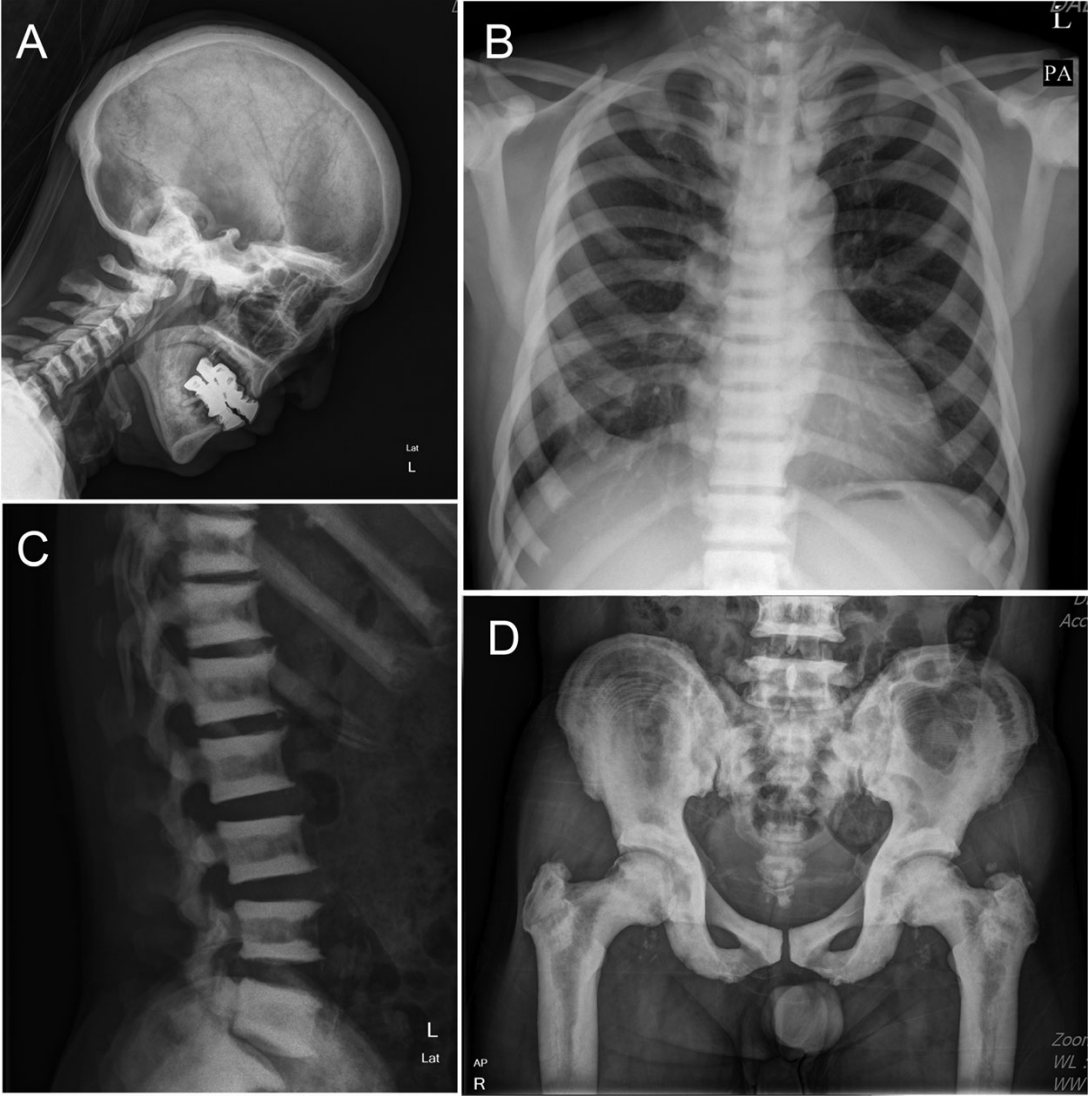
Skeletal radiographs reveal generalized symmetric increases in bone mass. (*A*) The cranium shows a thickening of the skull base. (*B*) Chest postero‐anterior radiograph shows the stark visualization of the anterior ribs. (*C*) Vertebral body shows a typical endplate sclerosis, called a “sandwich spine.” (*D*) Iliac crest also shows sclerotic and lucent bands, and the femoral bone shows cortical thickening and medullary encroachment.
